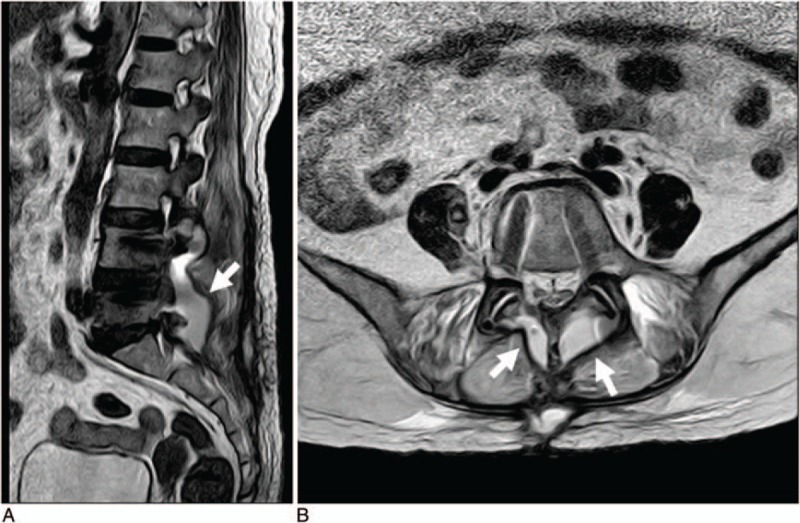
On the 19th day after the second operation, a large amount of cerebrospinal fluid leakage was noted on T2-weighted MRI. (A) Sagittal T2-weighted image showing a large amount of cerebrospinal fluid leakage in the lumbosacral space (arrow). (B) Axial T2-weighted MRI showing a large amount of cerebrospinal fluid leakage (arrows). MRI = magnetic resonance imaging.
